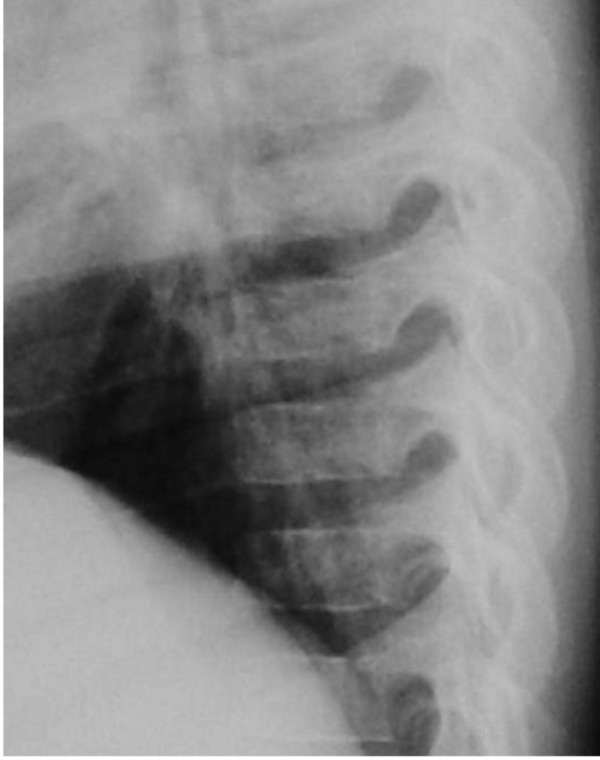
Lateral spine radiograph shows platyspondyly of the thoracic vertebral bodies associated with widening of the intervertebral spaces.
